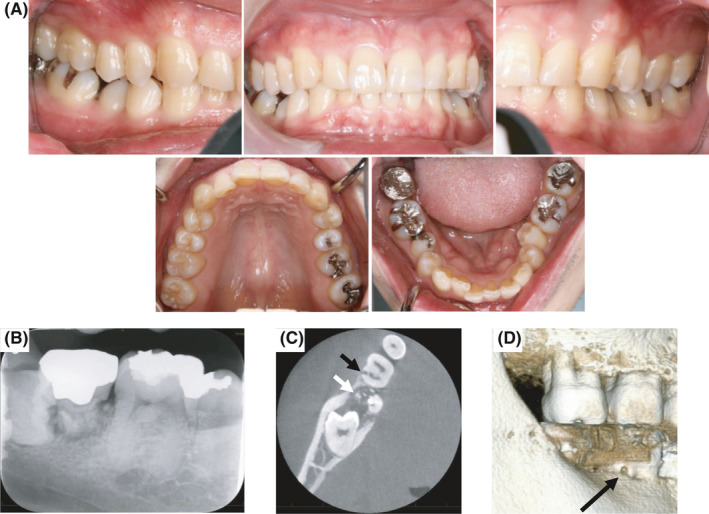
Photograph and radiogram of the oral cavity on the first examination. A, Photograph of the oral cavity, showing that the occlusion of the full cast crown of the right mandibular second molar had already been adjusted and the occlusal surface had become flat. B, Dental radiograph, indicating external root resorption in the right mandibular second molar. C, Axial view of cone‐beam computed tomography (CBCT), showing external root resorption in the right mandibular second molar (white arrowhead) and external cervical resorption on the buccal surface of the distal root of the right mandibular first molar (black arrowhead). D, Three‐dimensional reconstruction of CBCT, showing the resorption cavity on the buccal surface of the distal root of the right mandibular first molar (black arrowhead)
